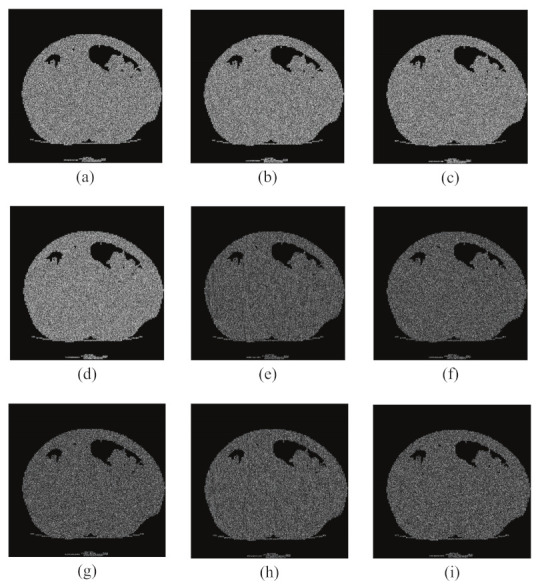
Key sensitivity test for CT Abdomen in encryption processes. (a) Encrypted image with KEY; (b) Encrypted image with KEY1; (c) Encrypted image with key KEY2; (d) Encrypted image with key KEY3; (e) difference between (a) and (b); (f) difference between (a) and (c); (g) difference between (b) and (c); (h) difference between (b) and (d); and (i) difference between (c) and (d).
